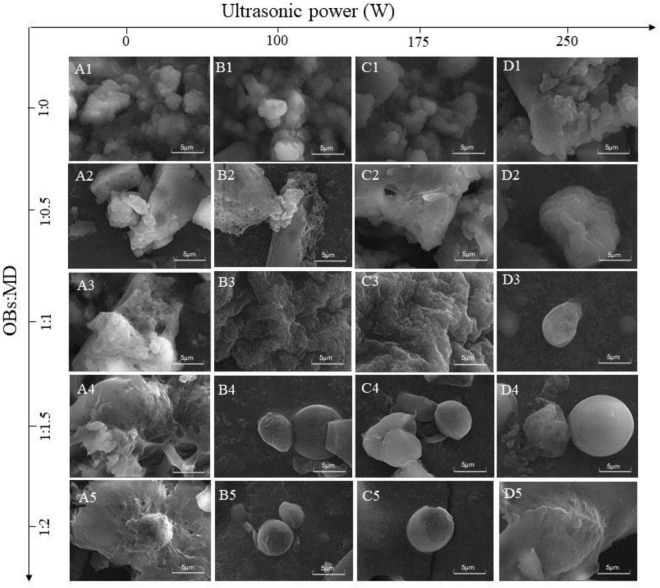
SEM images of OBs, OB-MD 0.5, OB-MD 1, OB-MD 1.5, and OB-MD 2 after different ultrasound power treatments. (A1–A5) No ultrasound, (B1–B5) 100 W ultrasound, (C1–C5) 175 W ultrasound, and (D1–D5) 250 W ultrasound.
